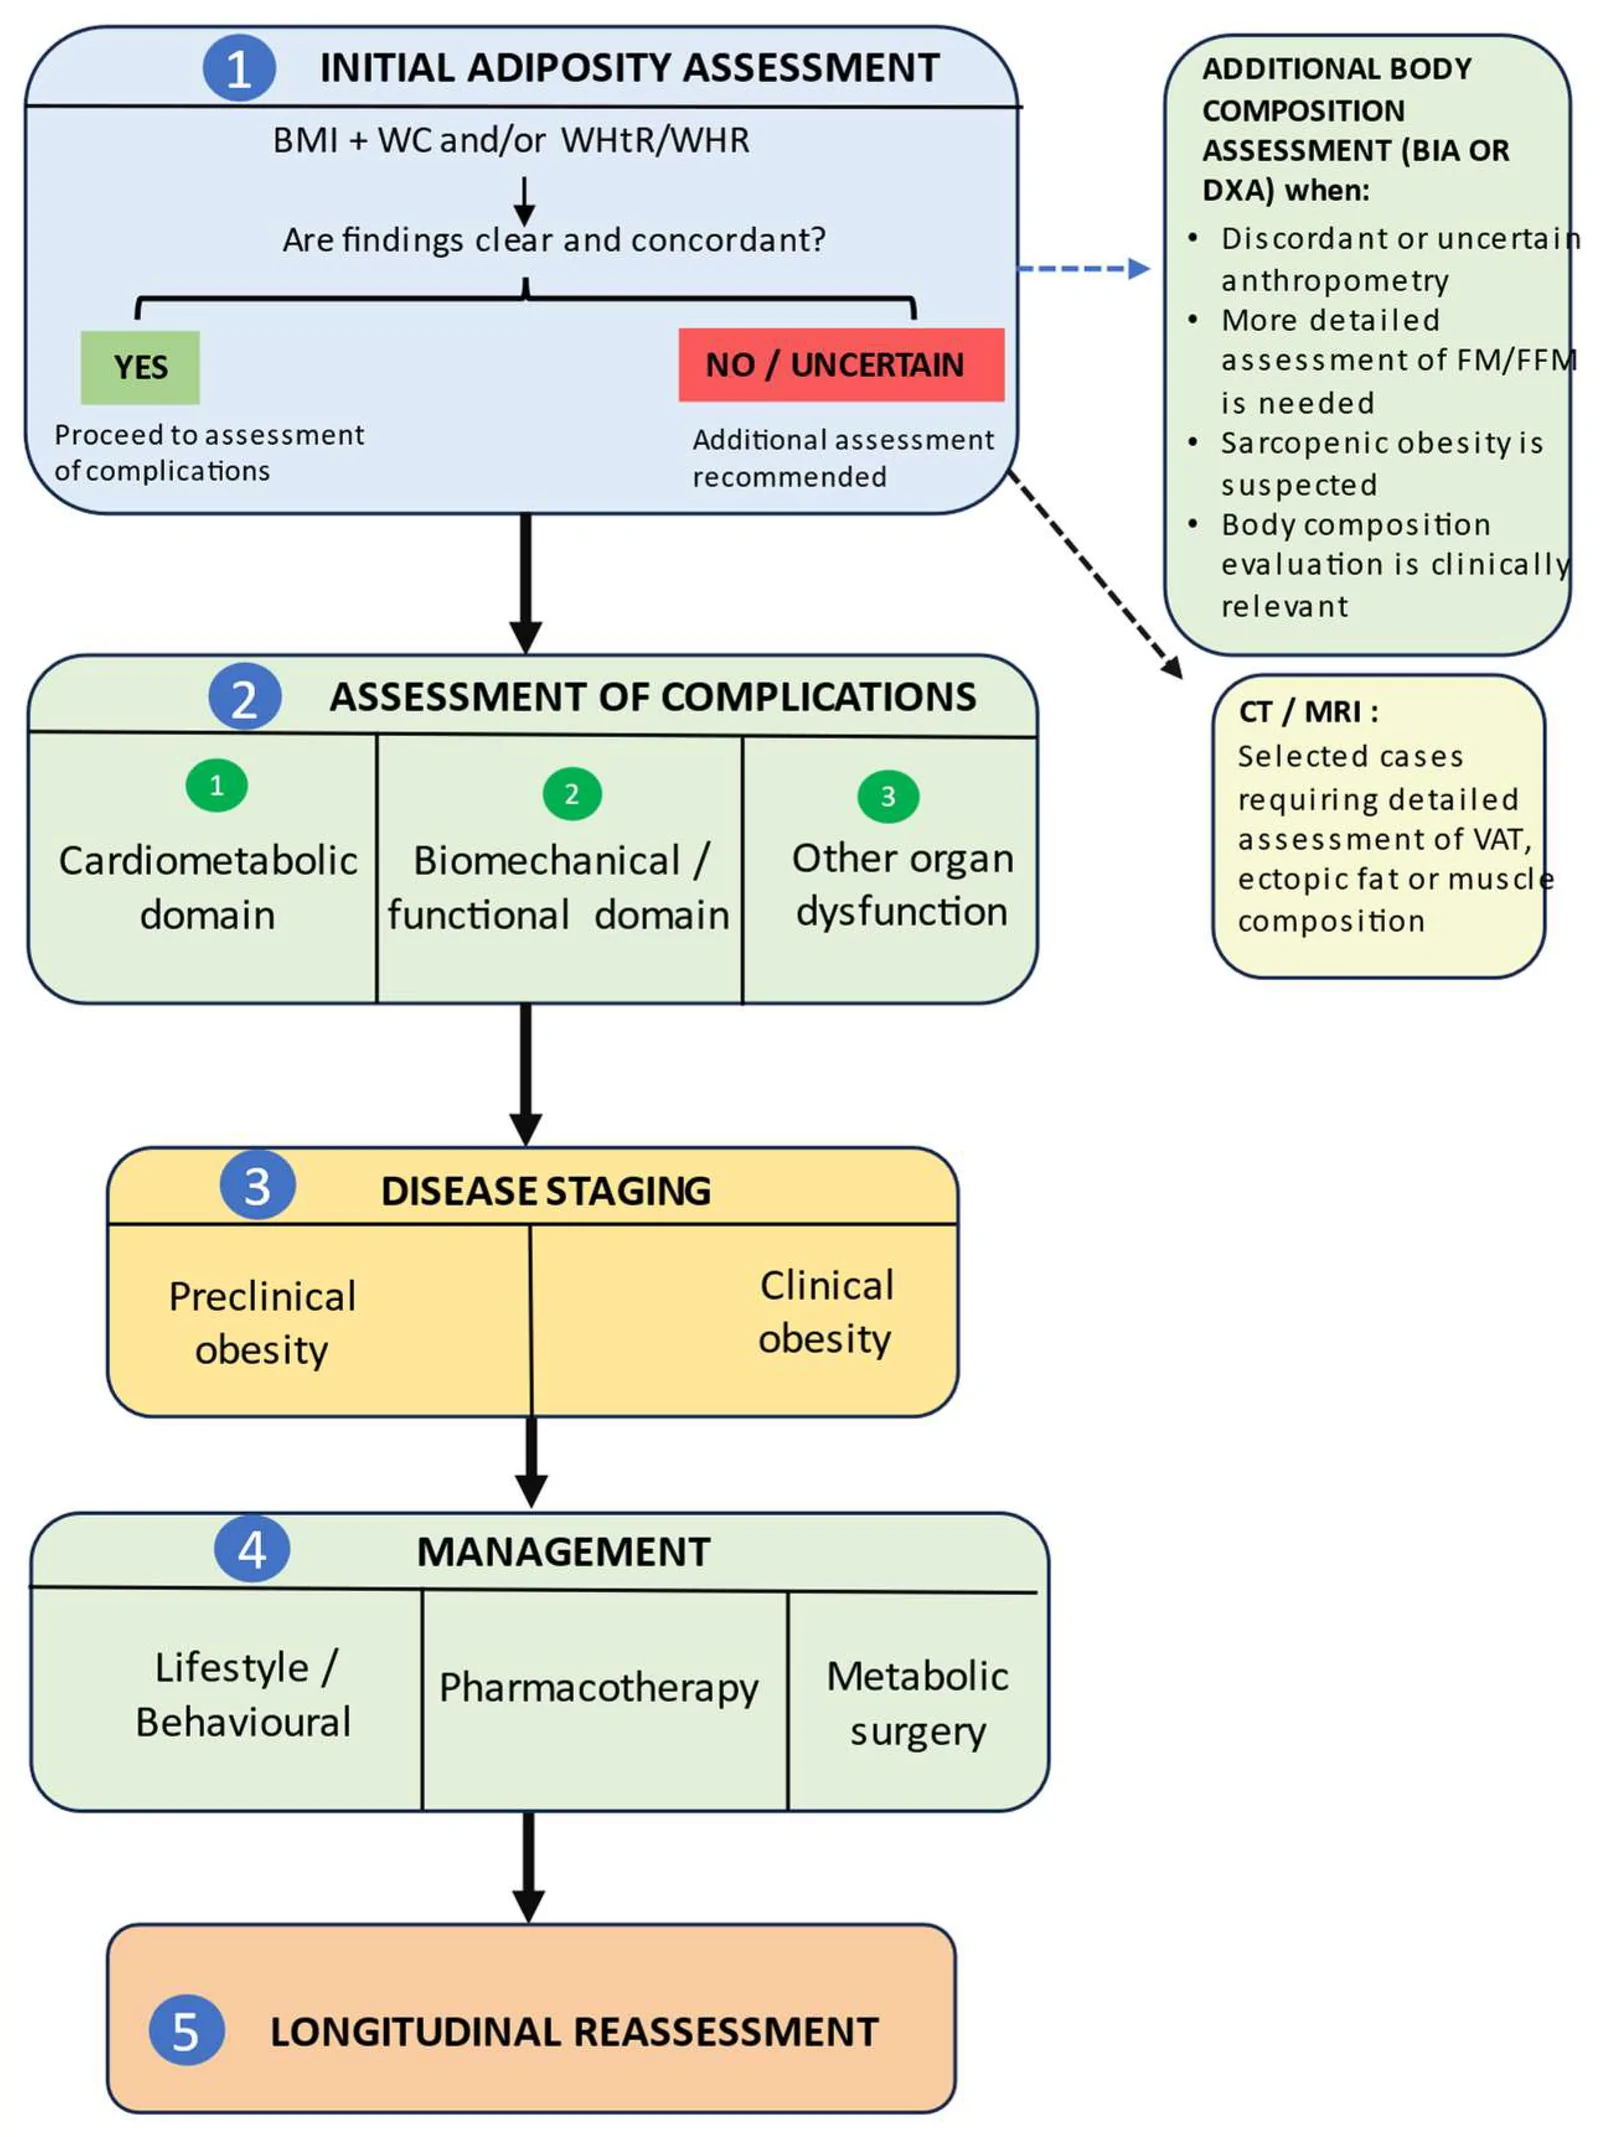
Practical clinical pathway integrating adiposity assessment, obesity complications, disease staging, management, and longitudinal reassessment. Abbreviations: BMI, body mass index; WC, waist circumference; WHR, waist-to-hip ratio; WHtR, waist-to-height ratio; BIA, bioelectrical impedance analysis; DXA, dual-energy X-ray absorptiometry; FM, fat mass; FFM, fat-free mass; VAT, visceral adipose tissue; CT, computed tomography; MRI, magnetic resonance imaging.
